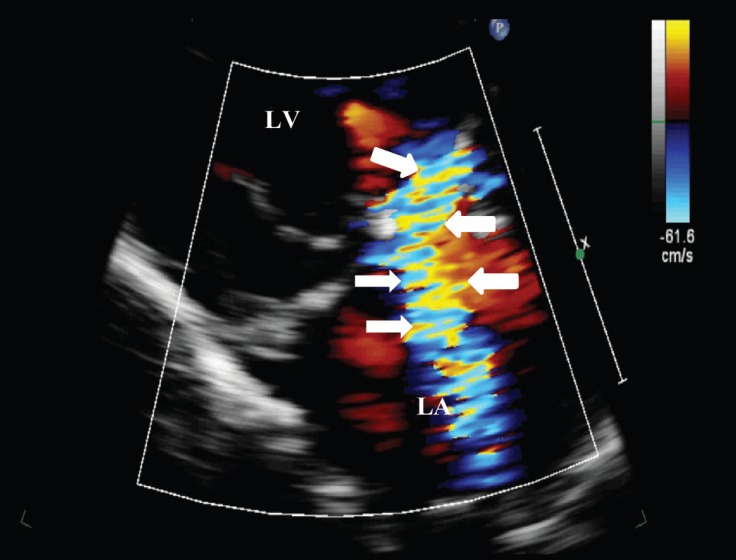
Transthoracic echocardiography view of the centrally directed mitral regurgitation jet by color Doppler (arrows).
